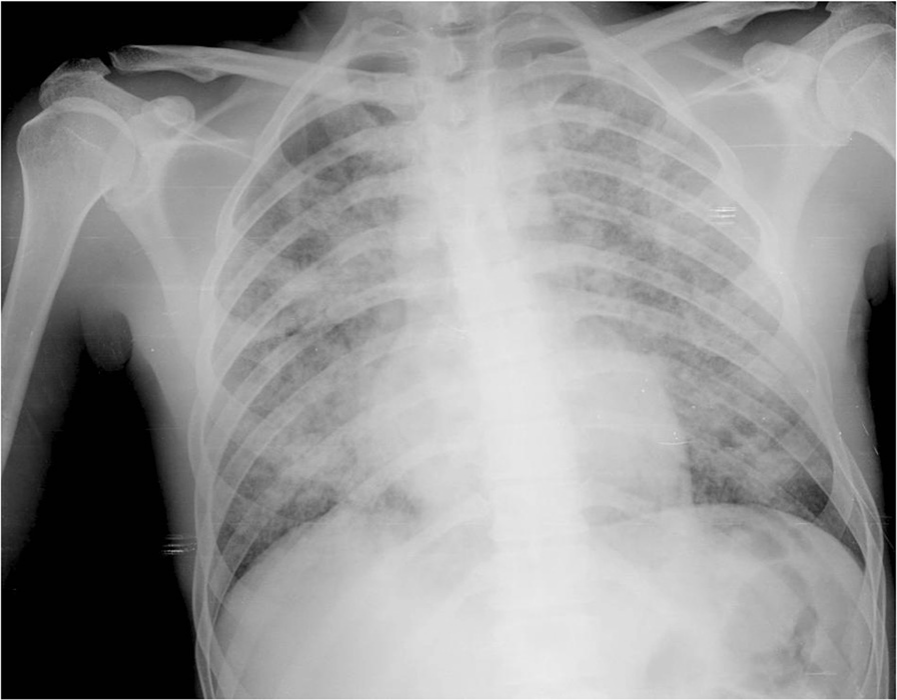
Chest X-ray performed at admission. The X-ray displayed bilateral pulmonary diffuse infiltrates
